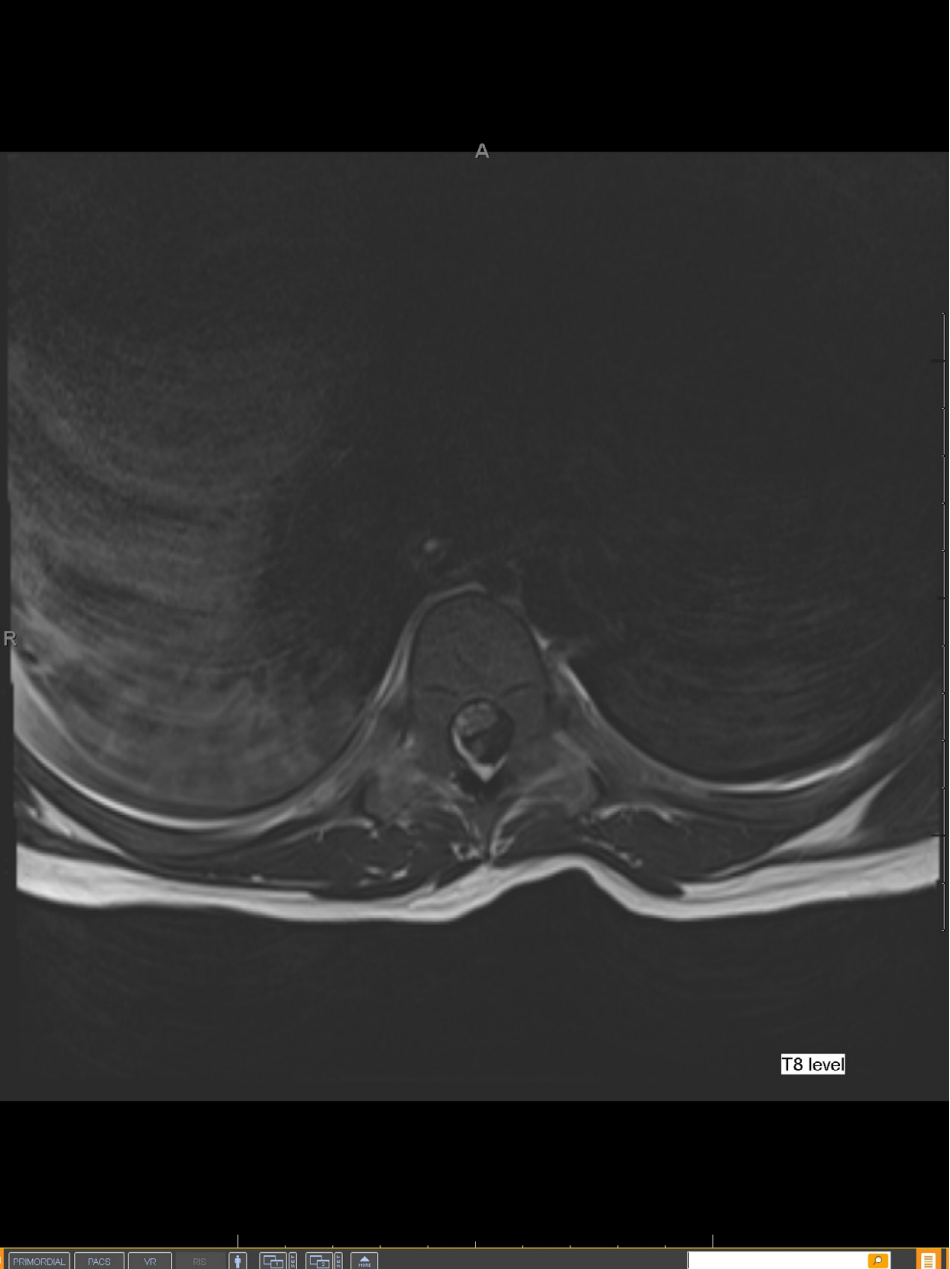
MRI axial image is showing a predominately T2 hypo‐intense lesion within the thoracic spinal canal at T8 level compressing the cord anteriorly and increased cord signal consistent with edema.
